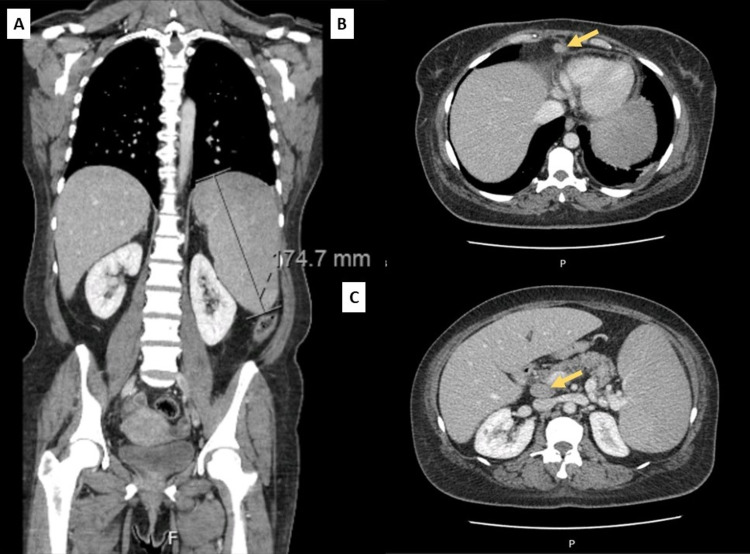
Cervical-thoracic-abdominal-pelvic CT scan A. Suggestive features of chronic liver disease, splenomegaly (17.5 cm), and signs of portal hypertension; discreetly prominent main bile duct (10 mm) secondary to cholecystectomy. B. Ganglion formation (18 x 11 mm) anteriorly to the pericardium. C. Several ganglia in the hepatic hilum (largest: 28 x 17 mm) CT: computed tomography
